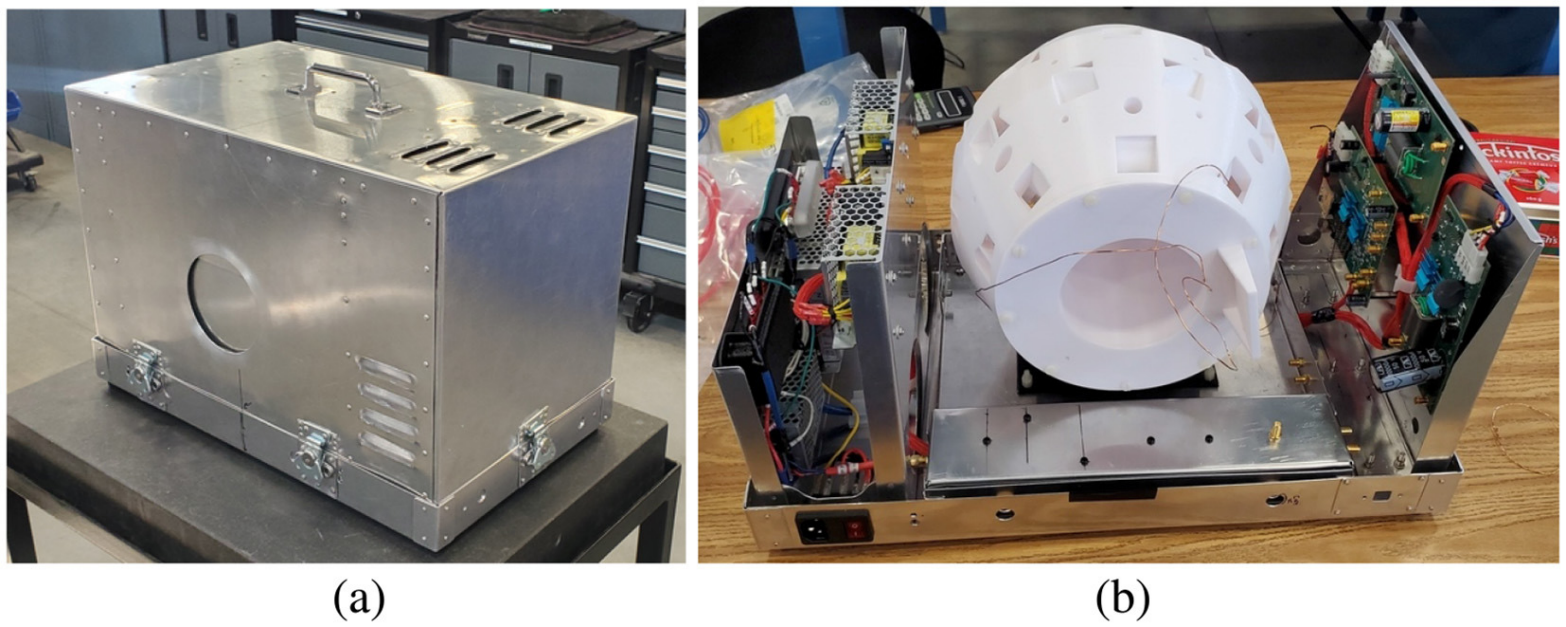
The *SIIT Owl MRI* that was built by the student team during the summer of 2024. (A) The completed MRI package. (B) A view of the MRI, with the covers removed and before the coils were finished and the magnet blocks installed.
